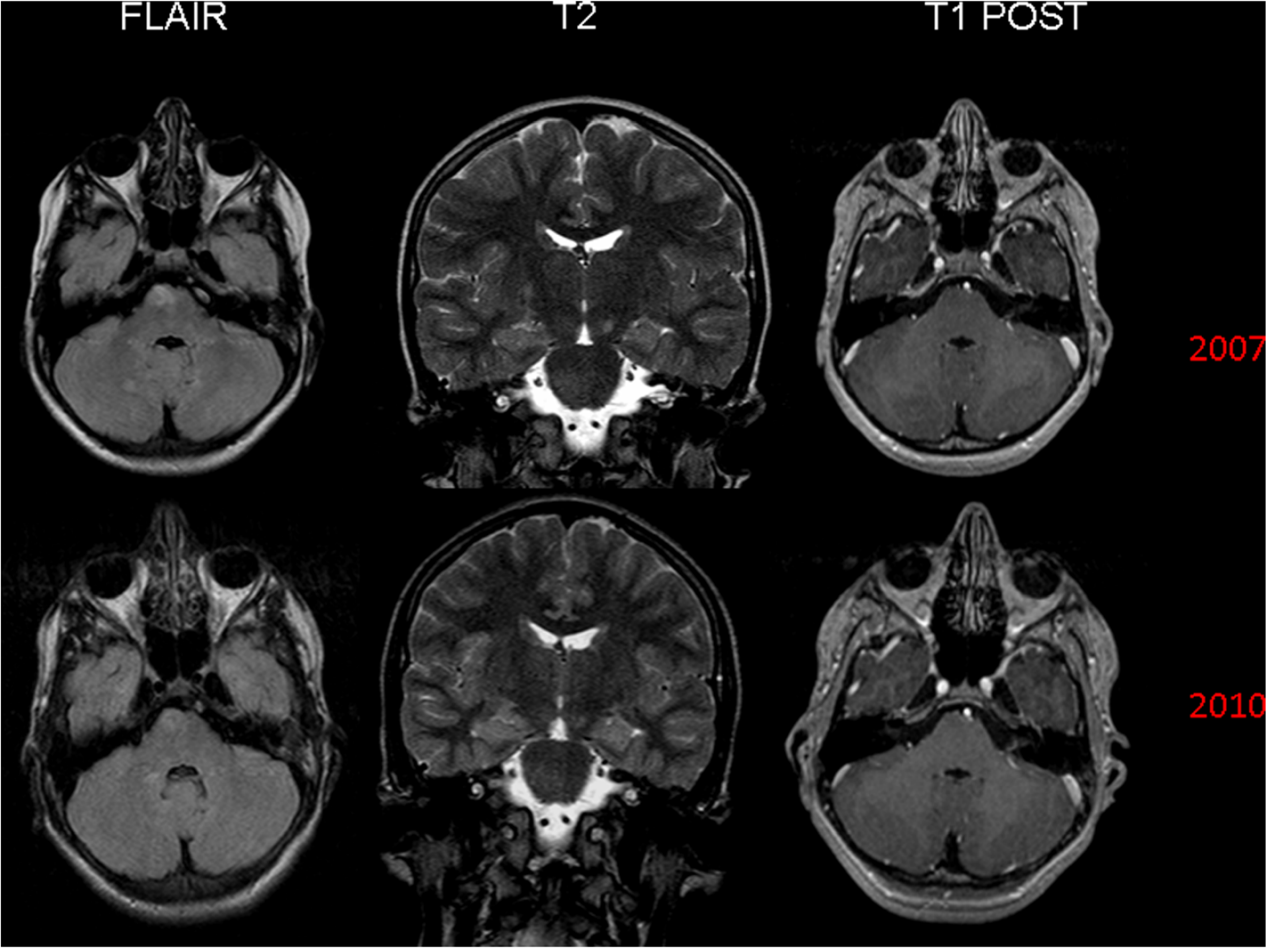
MRI (Flair, T2, and T1 sequences) performed in the child at the admission (2007) and four years later (2010). 2007: Hamartoma in the right pontine region and swallen aspect of the omolateral cortico-spinal tract. High signal intensity foci diffuse in the cerebellar emispheres (T2 hyperintensities). 2010: unmodified size of the pontine hamartoma and persistent bulge of the right cortico-spinal tract. Unchanged the UBOs lesions too.
